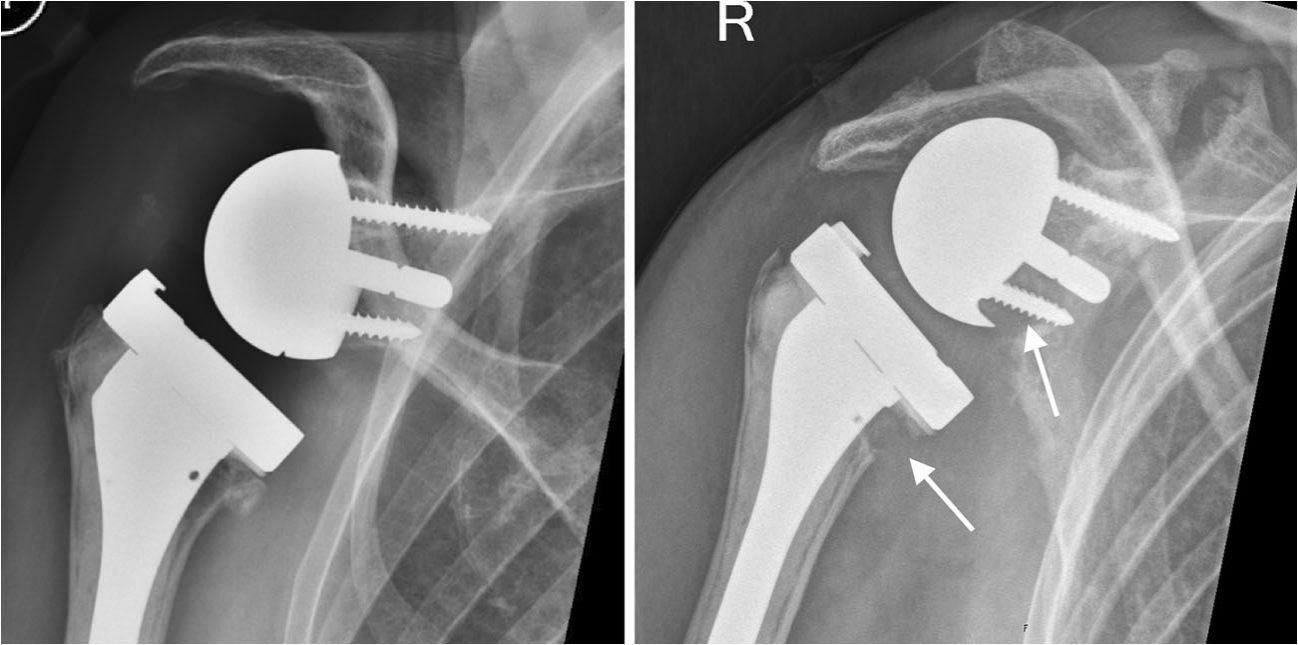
Anteroposterior radiographs of patient 8 showing an intact acromion 6 weeks postoperatively and a pseudarthrosis 18 months after a traumatic scapular spine fracture. Compensatory upward rotation of the scapula to avoid the subacromial conflict caused an inferior impingement with polyethylene wear, scapular notching and osteolysis of the calcar
